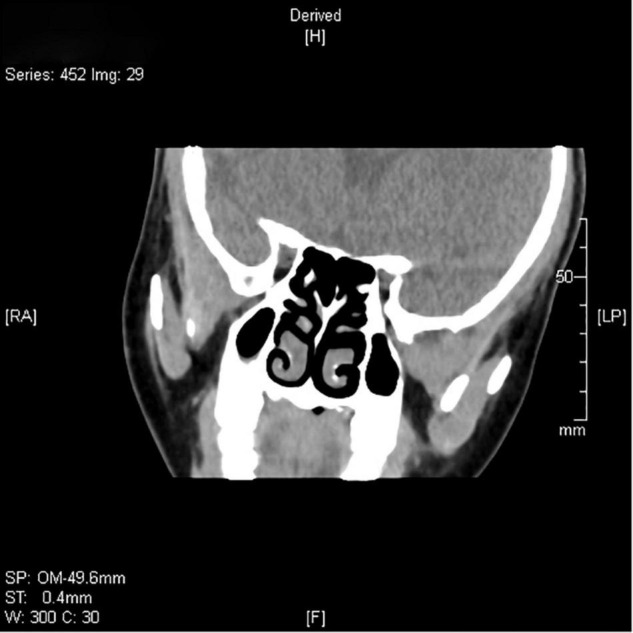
The paranasal sinus CT scan of the proband shows non-specific thickening of the mucosa on the bilateral maxillary sinuses.
